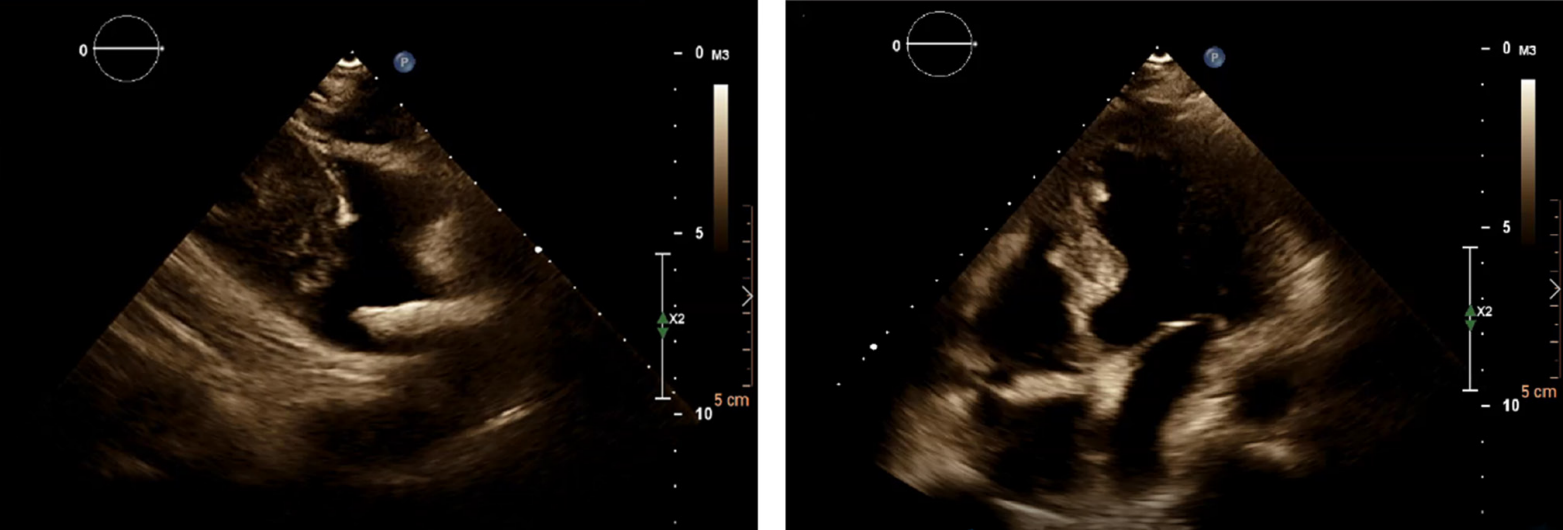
The transthoracic echocardiography of the right ventricular inflow in the four‐chamber view shows an elongated irregular hypermobile echodense mass in the right atrium.
